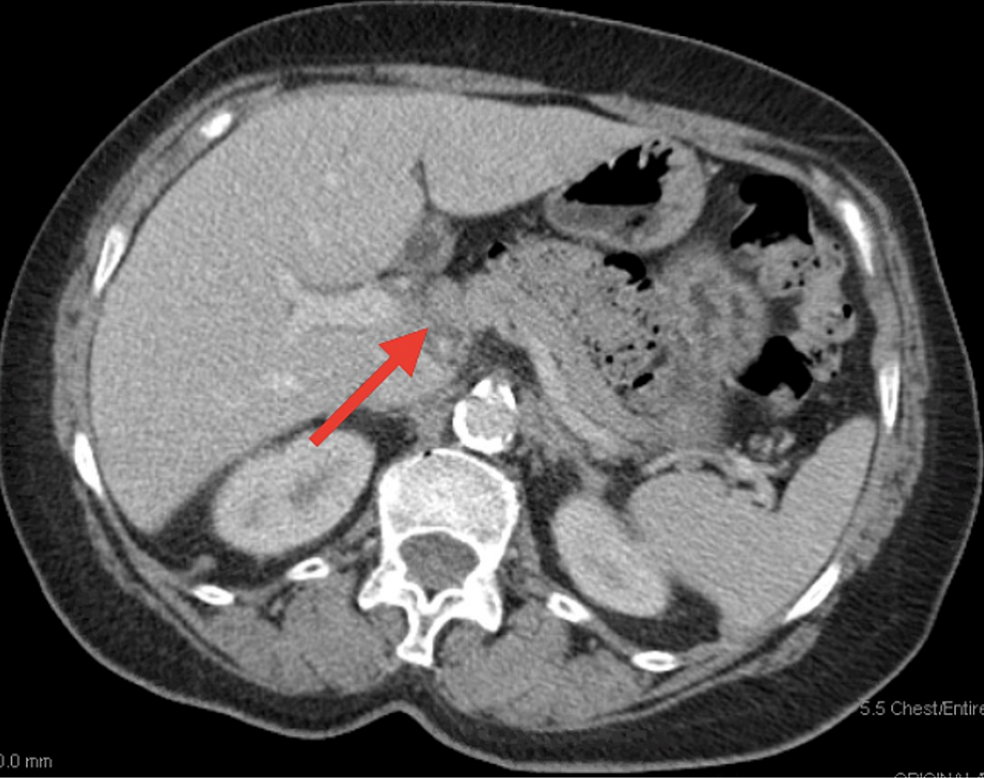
Spiral abdomen and pelvic CT demonstrating decreasing metastatic lymph node within gastrohepatic ligament shown with a red arrow.
